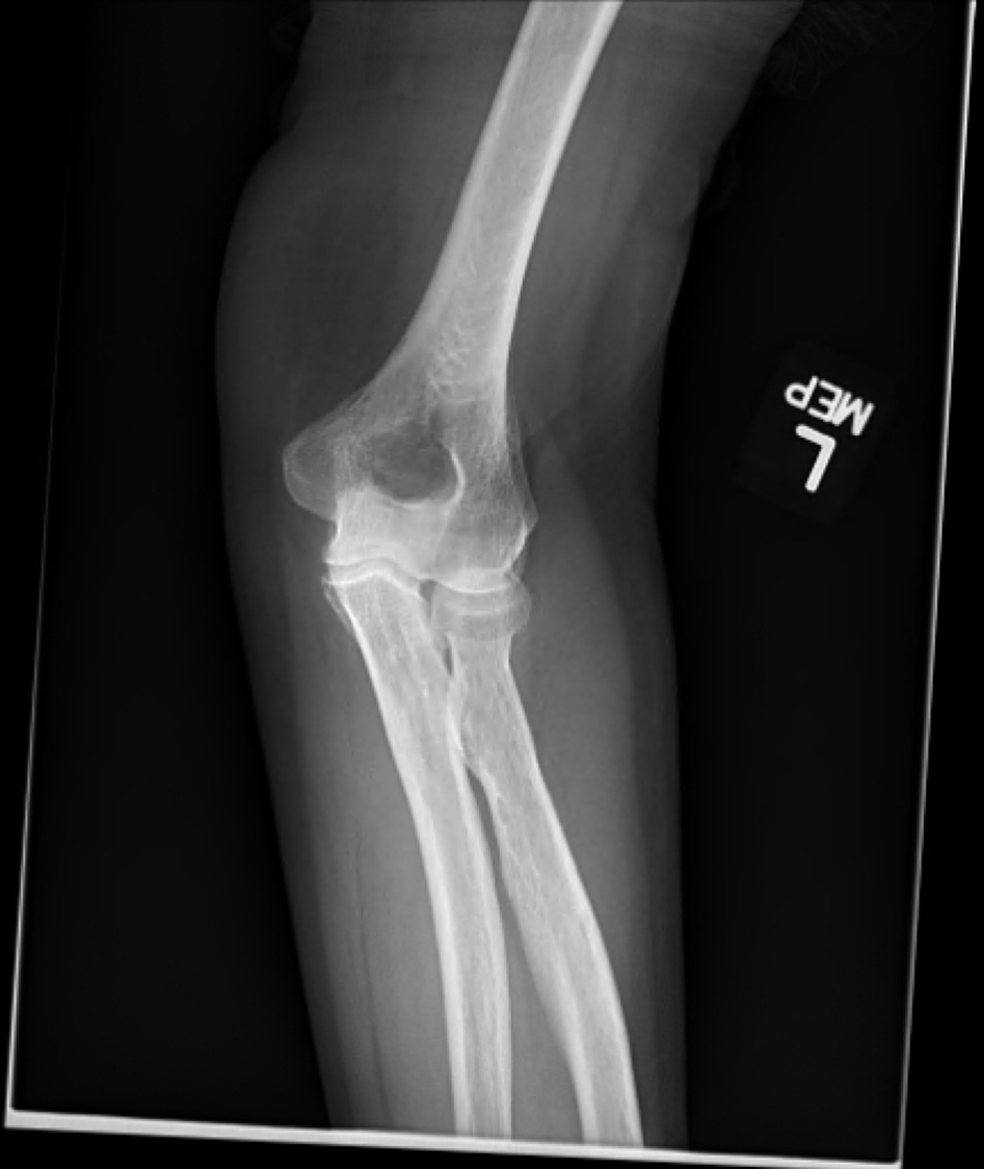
X-ray of patient's left elbow showing mild spurring around the head of the radius with no loose bodies noted.
